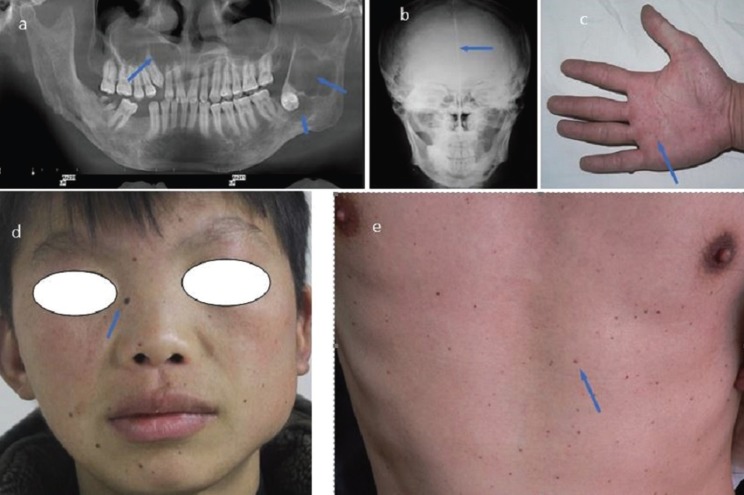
Some of the clinical manifestations of nevoid basal cell carcinoma syndrome. Presence of multiples synchronous odontogenic keratocysts in the maxilla and mandible with unilocular pattern involving third impacted molar (a). Antero-posterior skull radiograph revealing calcification of the falx cerebri (b), multiple palmar pits (c). Multiple cutaneous nevi located in all facial and thoracoabdominal region (d, e).
